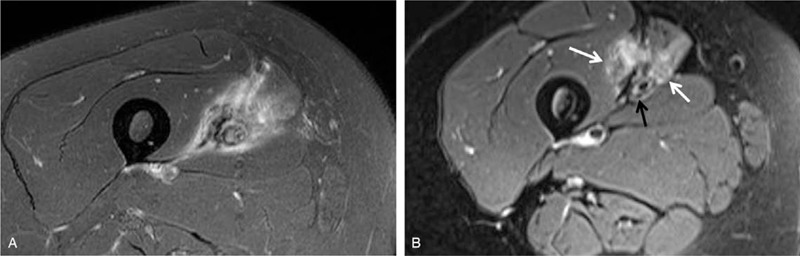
Magnetic resonance (MR) imaging performed at the general surgery clinic 1 year before referral and at 12-month follow up. (A) Narrowing of the artery could be seen in T2-weighted image. The lesion showed an ill-defined heterogeneous signal and severe perilesional edema. (B) MRI T2-weighted imaging at 12 months still showed an ill-defined lesion while the edema was reduced (white arrows). It was not possible to clearly differentiate the vein due to vessel collapse (black arrow).
